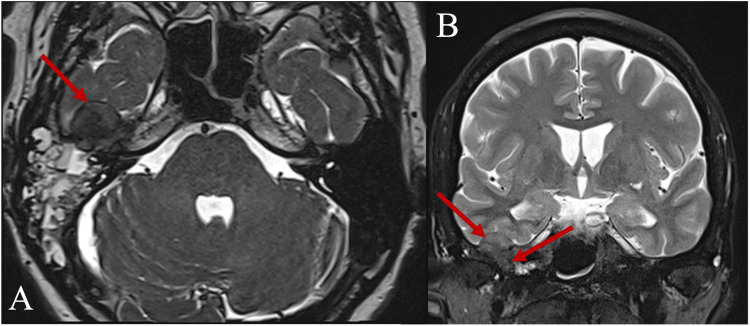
Head MRI before the latest surgery, T2-weighted axial (A) and coronal (B) views Tumor recurrence is seen. Arrows show intracranial infiltration into the dura mater of the temporal fossa (~18 mm in length and ~8 mm in width segment) and subtemporal extension of the tumor. Clear invasion into brain parenchyma or any compression of its structures is not observed.
